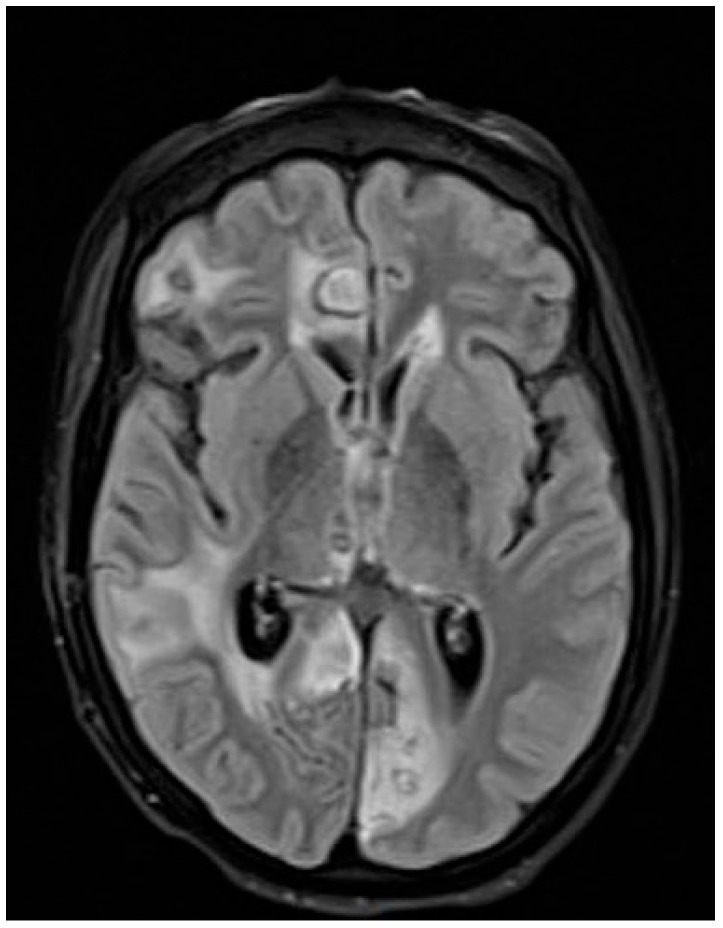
Brain MRI two months after therapy.
